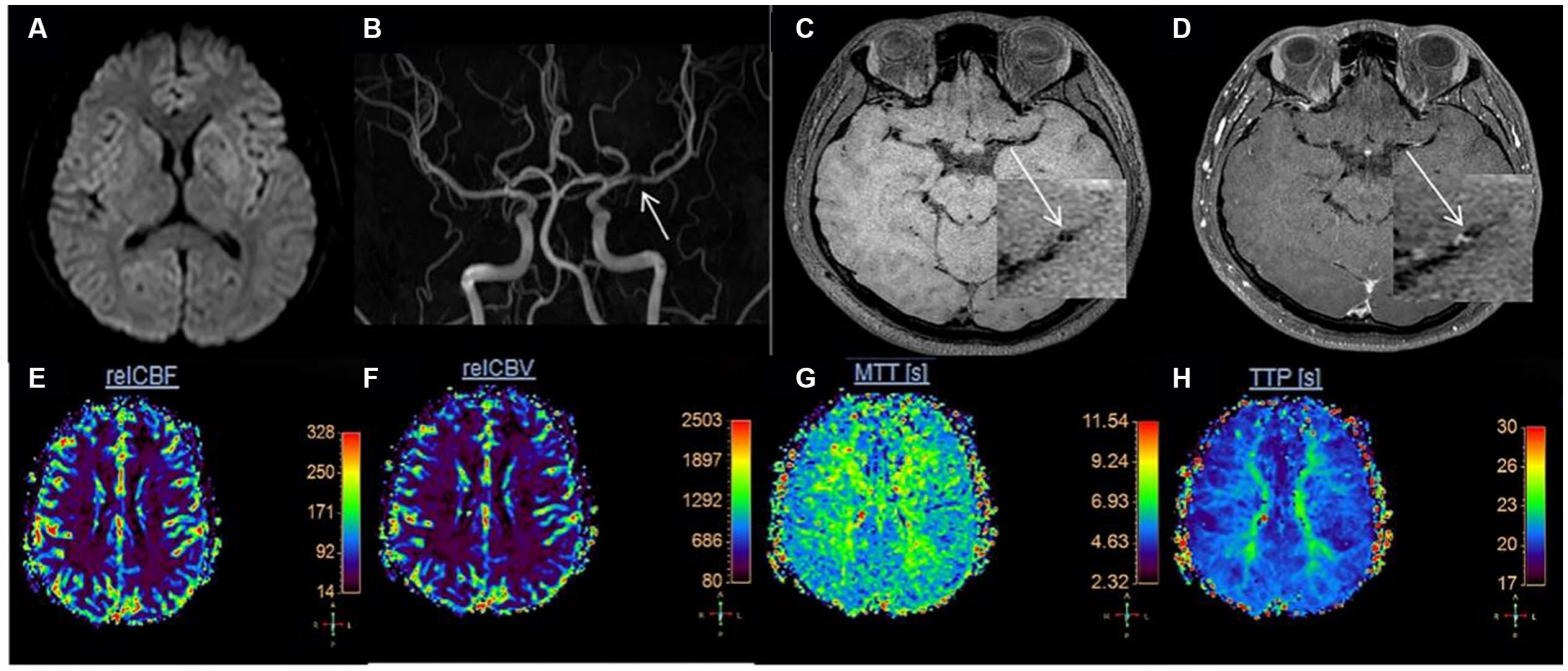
Measurement of vessel and plaque characteristics in non-hypoperfusion. Female, 59 years old, left upper limb weakness for more than 3 h. (A) DWI is normal; (B) The stenotic site of the left MCA. The white arrow shows the stenotic site of the left MCA; (C) T1-weighted HR-VWI imaging shows the plaque in the left MCA. The white arrow shows the short-axis view; (D) Enhanced T1-weighted HR-VWI imaging showing the measurement at the most narrowed site in left MCA. OWA = 5.19 mm^2^, IWA = 1.33 mm^2^. (E–H) Pseudo-color maps of rCBF, rCBV, MTT, and TTP were shown respectively, and none of them revealed the region of hypoperfusion. DWI, diffusion-weighted imaging; MCA, middle cerebral artery; HR-VWI, high-resolution vessel wall imaging; OWA, outer wall area; IWA, inter wall area.
